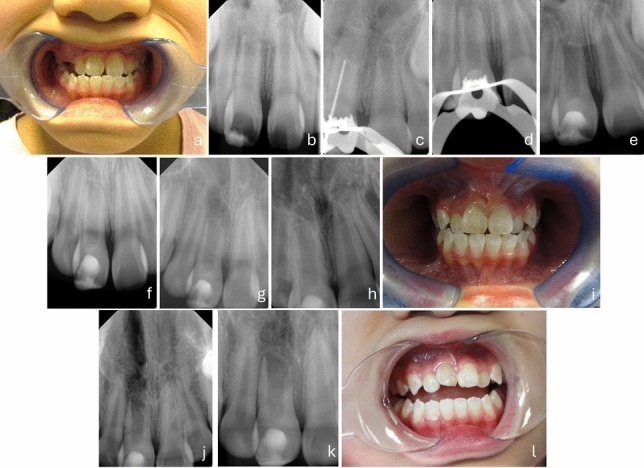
Pre-operative clinical and radiographical findings of tooth 11: a clinical and b radiographical appearance at the time for examination at the Department of paediatric dentistry at Eastman Institute, Public Dental Health Services, Stockholm, Sweden. Intraoperative and immediate postoperative radiographs of tooth 11: c establishment of the working length; d placement of the resorbable collagen scaffold and bioceramic material; e immediate postoperative radiograph and final restoration; f–i 2-year follow-up of tooth 11; j–l 3-year follow-up of tooth 11
